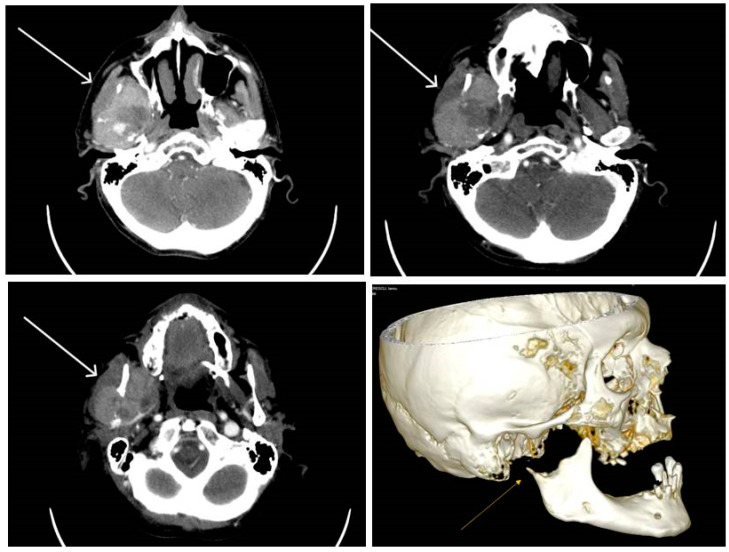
Computed tomography imaging—heterogeneous tissue mass with necrotic areas at the level of the right masticatory space (arrow) with lyses of the mandibular condyle.
